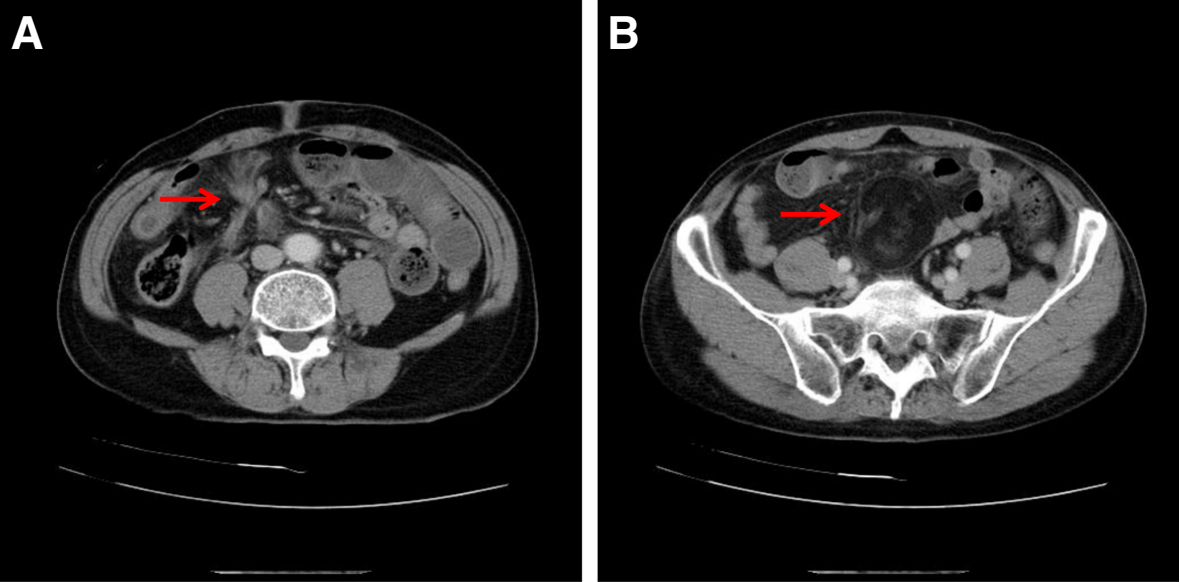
A contrast-enhanced computed tomography scan of the abdomen. a The twisted small intestine (*arrow*). b A well-capsulated homogeneous mass (*arrow*)
